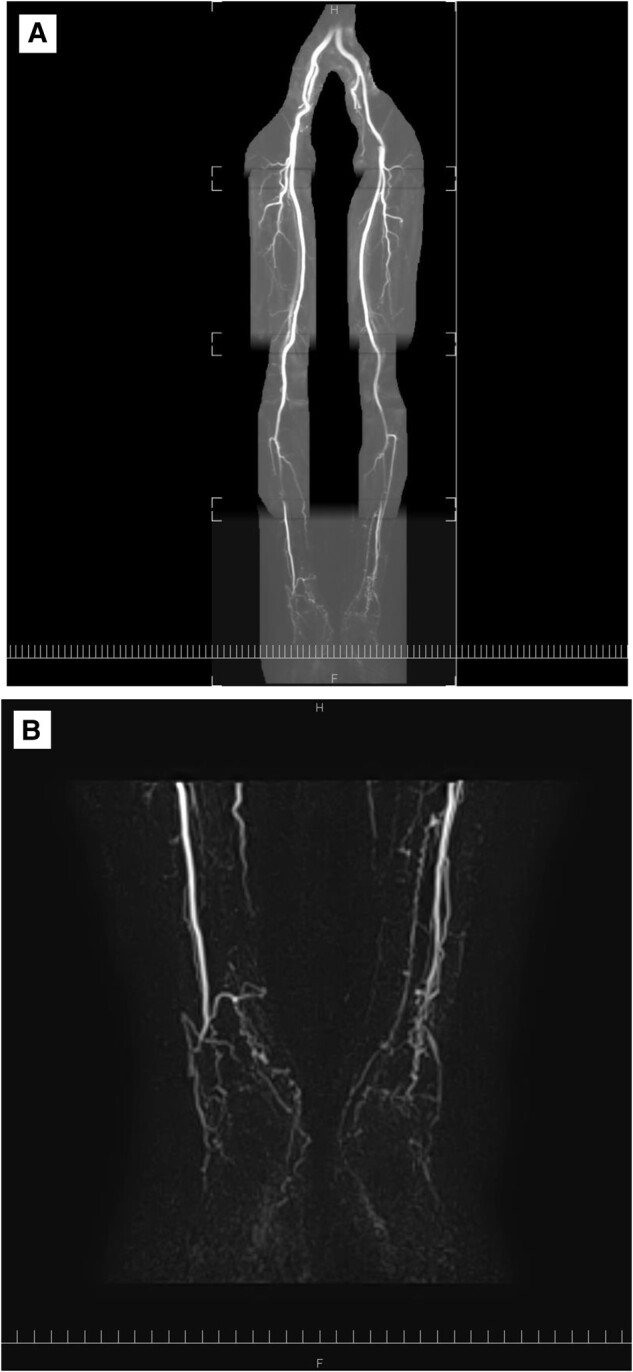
Non-contrast magnetic resonance angiography after 1 year of low-intensity pulsed ultrasound treatment. (*A*) No disruption of blood flow is seen within the imaging range. (*B*) Tortuosity and irregularity of blood vessels are noticeable in both feet.
